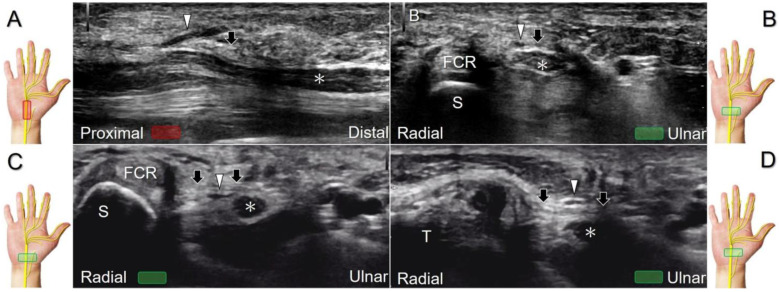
Sonographic imaging of the preligamentous type of recurrent motor branch of the median nerve. It divides from the median nerve proximal to the carpal tunnel as seen in the long-axis view (A), and resides superficial to the flexor retinaculum, as seen in the short-axis view (B), whereas the transligamentous type of recurrent motor branch accompanies the median nerve into the carpal tunnel (C), penetrating the retinaculum at the outlet of the carpal tunnel (D). Asterisk: median nerve; arrowheads: recurrent motor branch of the median nerve; arrows: flexor retinaculum. FCR: flexor carpi radialis tendon.
